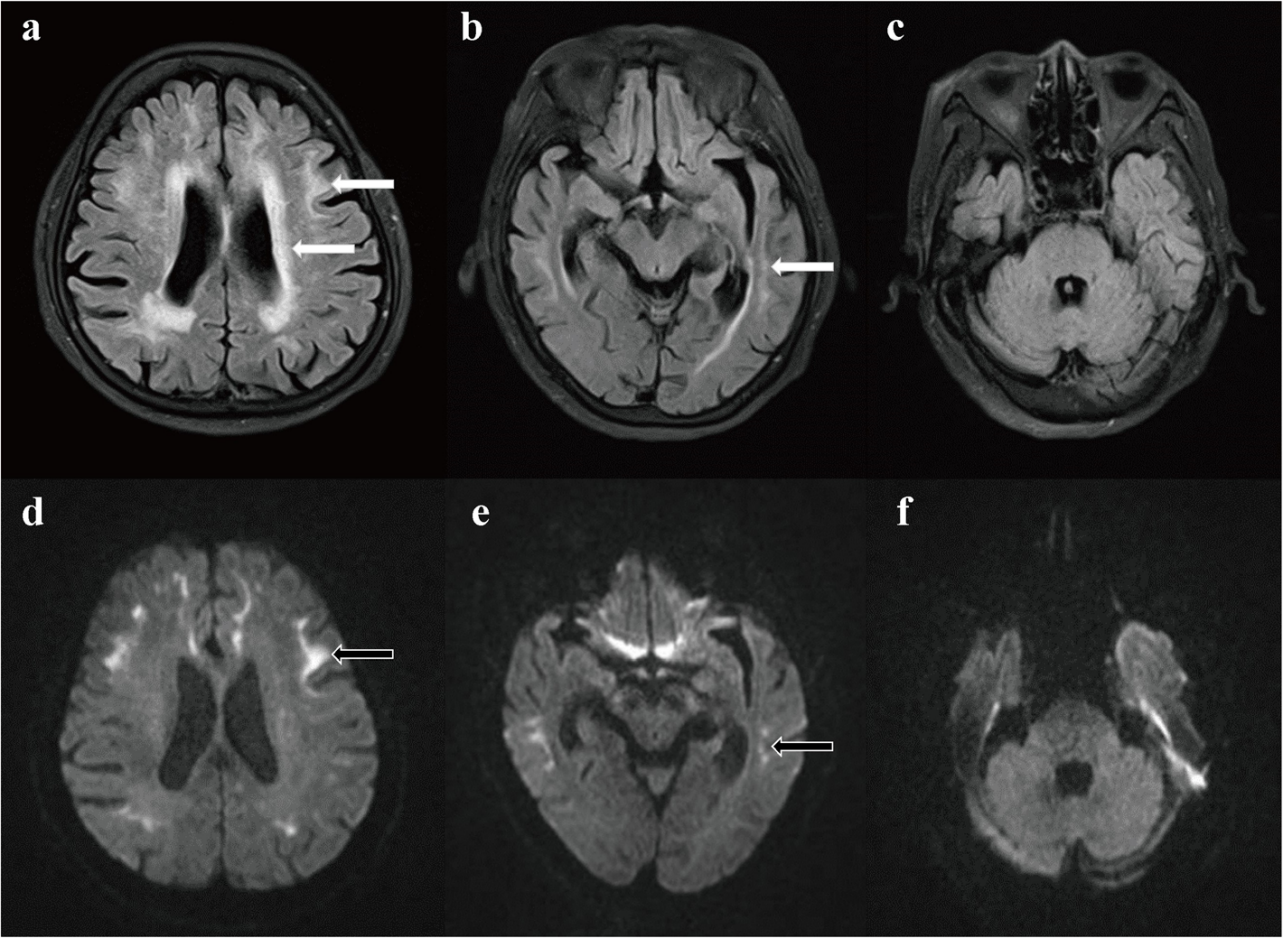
a-f: Cranial MRI performed 3 months after onset. a-c: Leukoencephalopathy was evident on the T2 FLAIR images. A high-signal intensity in the white matter of the cerebral hemisphere, especially at the subcortex of the frontotemporal and corona radiata, was found on the T2 FLAIR images (arrow). d-f: The DWI showed a symmetrically distributed strip-shaped hyperintensity of the corticomedullary junction in the bilateral frontal, parietal, and temporal lobes (arrow)
